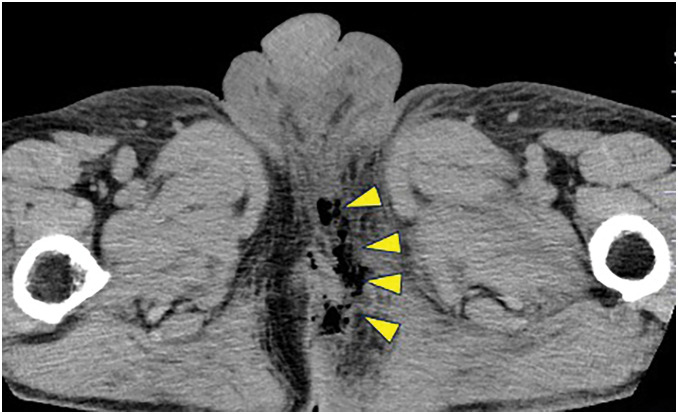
Initial plain CT findings. Edematous changes with gas formation in the soft tissues were observed on the left side of the anus (yellow arrowheads).
